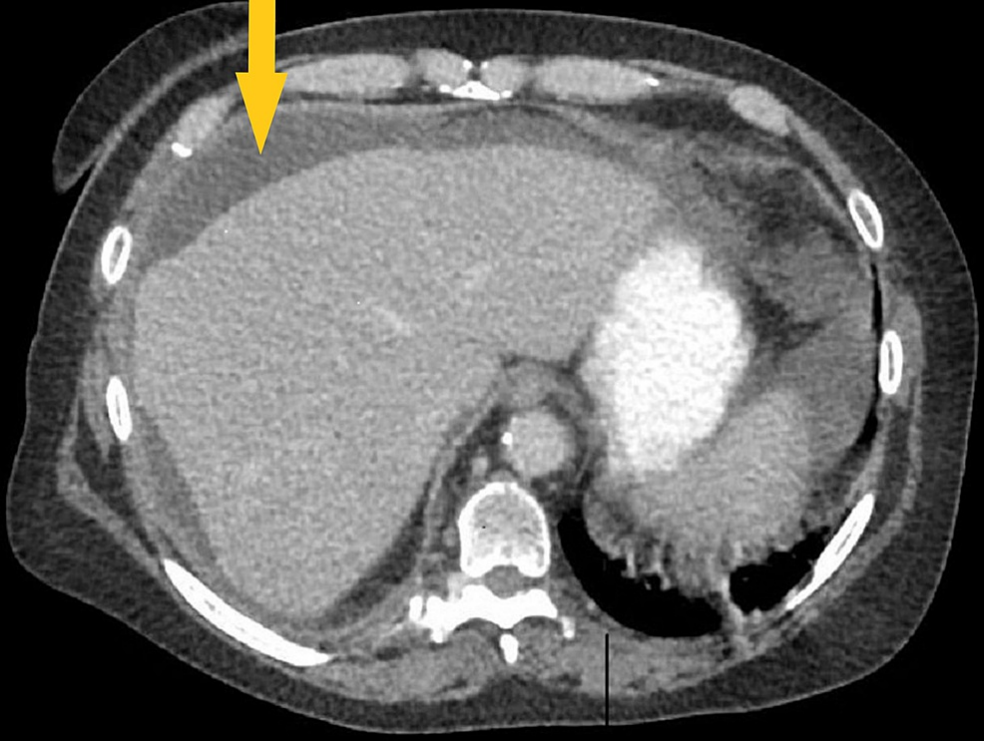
Computed tomography scan of the abdomen and pelvis with a loculated fluid collection measuring 17.8 × 13.4 × 16.7 cm, which is perihepatic bile ascites that has formed an extrahepatic biloma distorting the hepatic parenchyma.
